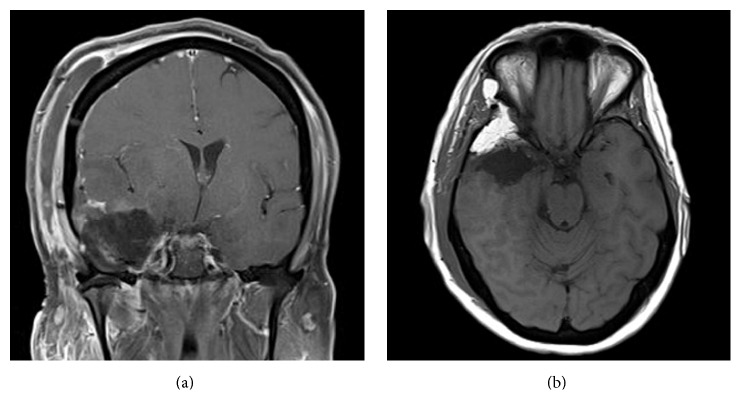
Postoperative T1-weighted gadolinium-enhanced MRI of the brain. Both (a) coronal and (b) axial sequences confirm gross total resection of all enhancing tumor. New hyperintensity appreciated at the anterior right temporal pole identifies abdominal fat graft placed during reconstruction.
